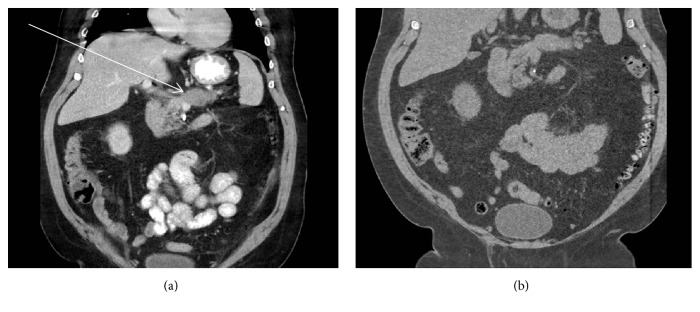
(a) Computed tomography scan with intravenous contrast, which showed 5.0 × 2.7 cm irregular, ill-defined, and hypoattenuating mass located in the pancreatic tail and body (labelled as thin arrow). (b) The prior noncontrast computed tomography scan four months earlier showed no lesions in the pancreas and abdomen.
